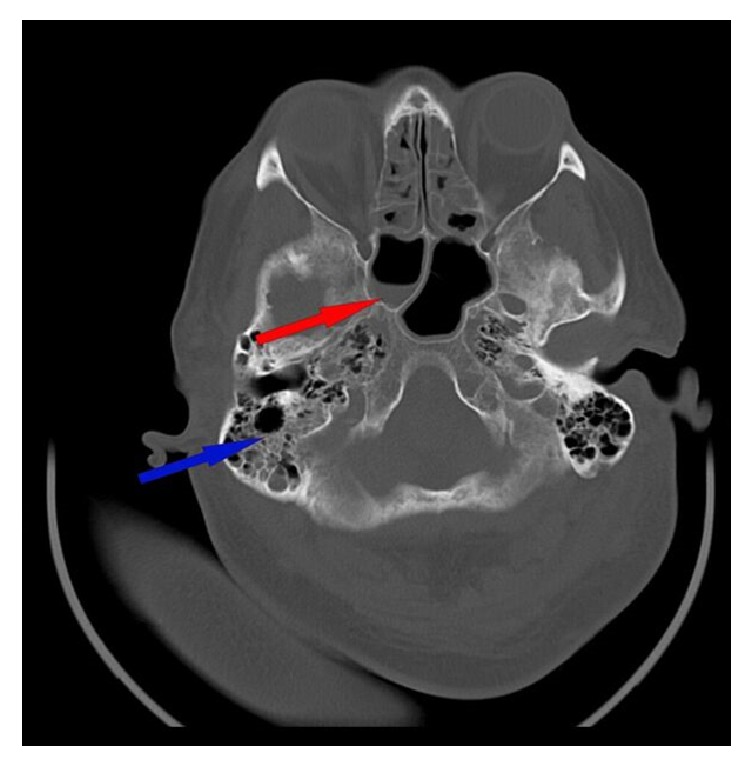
MR imaging of the cranium with bilateral mastoiditis (blue arrow) and sphenoidal sinus with air-fluid level (red arrow). In addition, polypoid swelling of mucous in both ethmoid sinuses.
